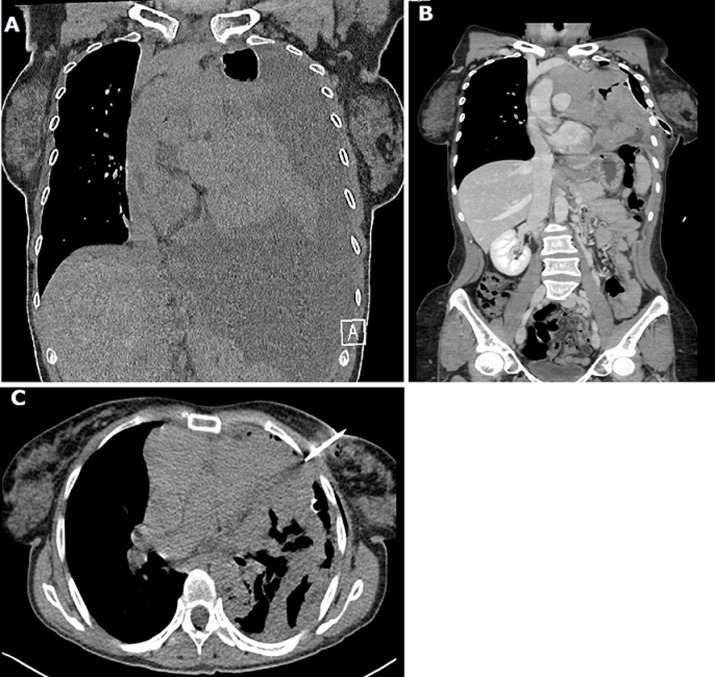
A) thoracic computed tomography (CT) scan demonstrating a giant mediastinal mass associated with massive left pleural effusion; B) post-28 Fr thoracic drainage demonstrating the extension of giant mediastinal mass; C) FNA thoracic CT scan biopsy
